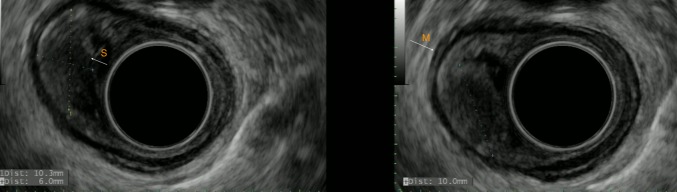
Endoscopic ultrasonography images revealing a well-demarcated, hypoechoic, homogenous lesion arising from the submucosal layer as depicted by white arrow (S). M represents muscularis propria.
